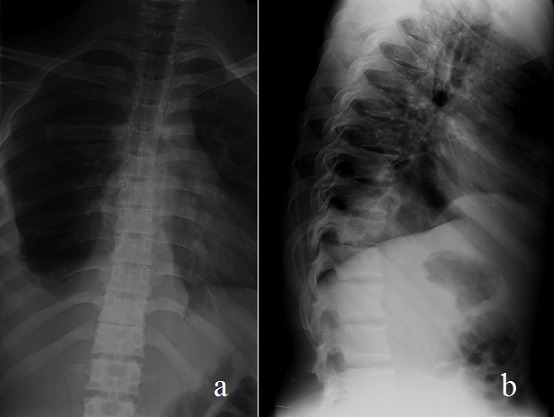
Radiographie du rachis dorsolombaire en incidence de face (a) et de profil (b) montrant un pincement de l'interligne intervertébrale T10-T11 avec destruction vertébrale en miroir, un fuseau paravertébral bilatéral et une angulation à faible courbure
